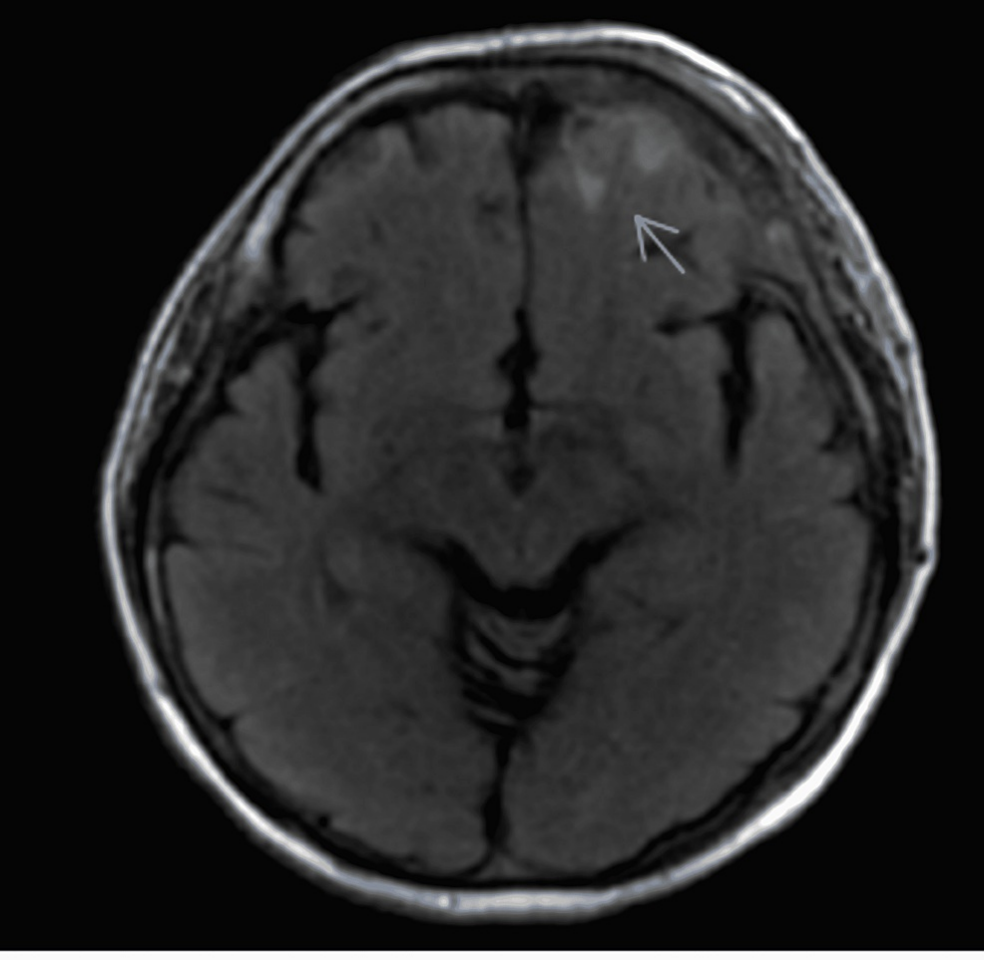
MRI showing a small hyperintense lesion in the left frontal cortical region. MRI: magnetic resonance imaging
